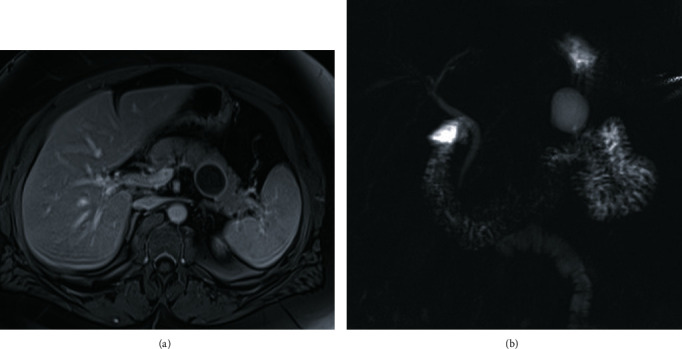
(a) Axial post contrast MRI demonstrates a 3.3 cm pancreatic body/tail cystic lesion without enhancing nodules. (b) MRCP demonstrates a pancreatic body cystic lesion with no discrete connection with the pancreatic duct and a normal pancreatic duct diameter.
